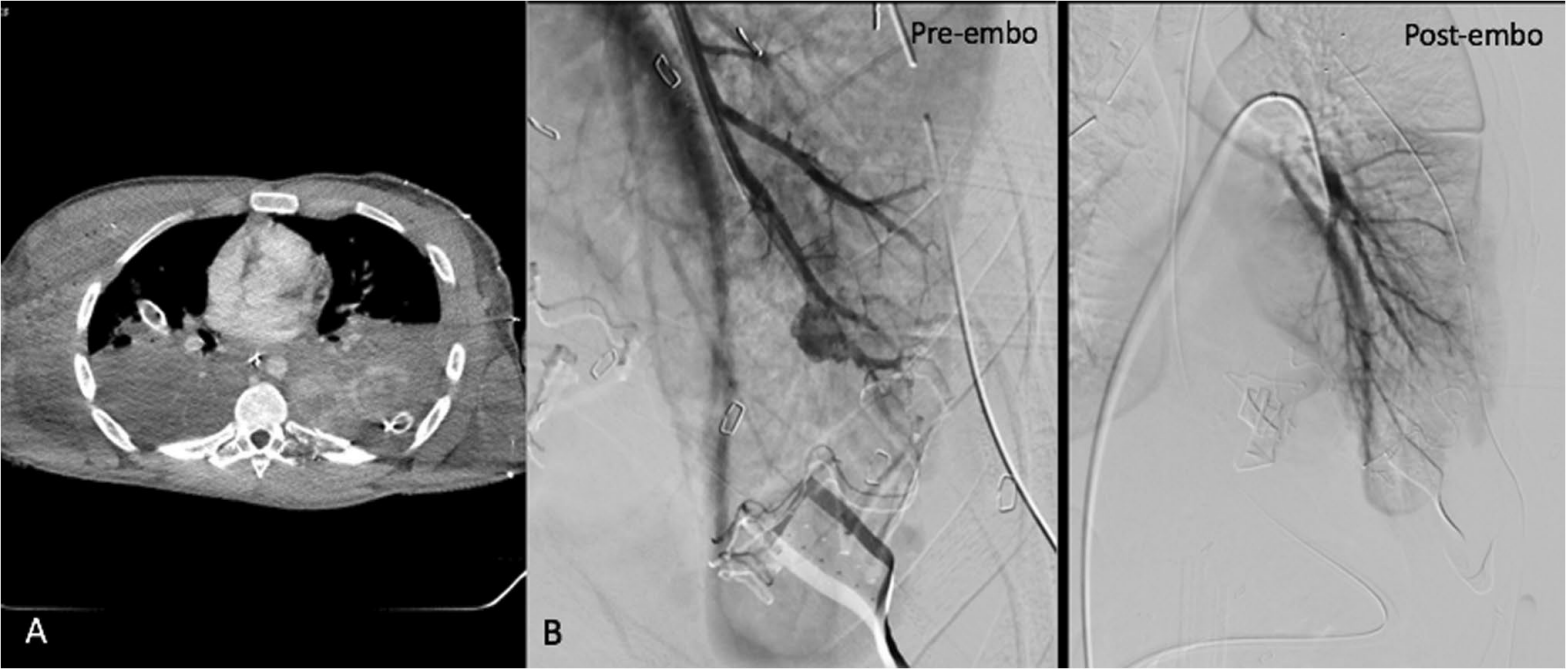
CT chest (A) and pre and post pulmonary angiography (B) of case 3
